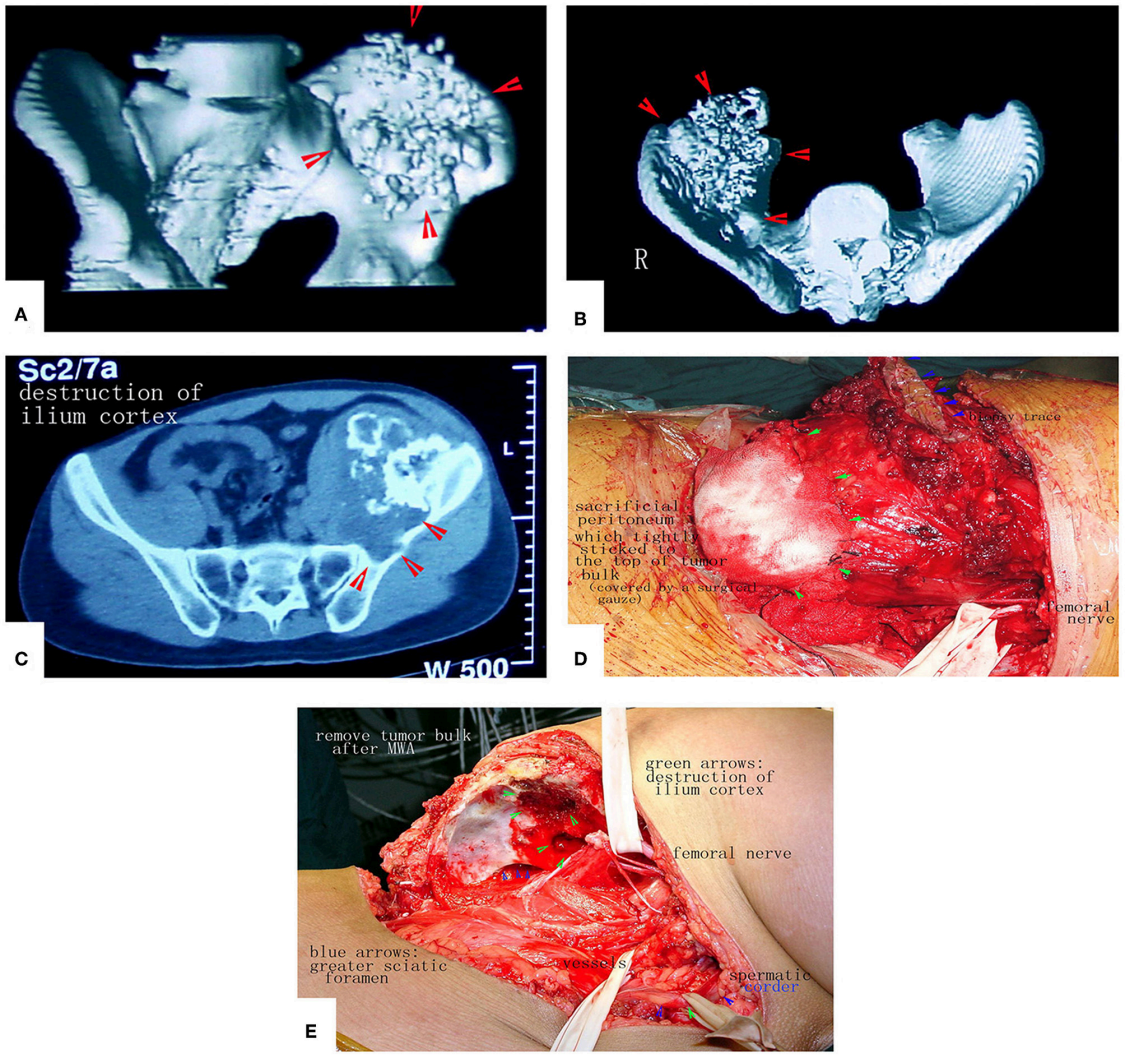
A patient with a massive pelvic chondrosarcoma was treated by MWA. (A–C) Images of the tumor. CT scan showed huge tumor of pelvis. (D) Due to the strict adhesion between the peritoneum and tumor bulk, a portion of the peritoneum that adhered tightly was sacrificed to prevent tumor cell contamination. (E) The tumor mass was removed. No reconstruction was needed.
